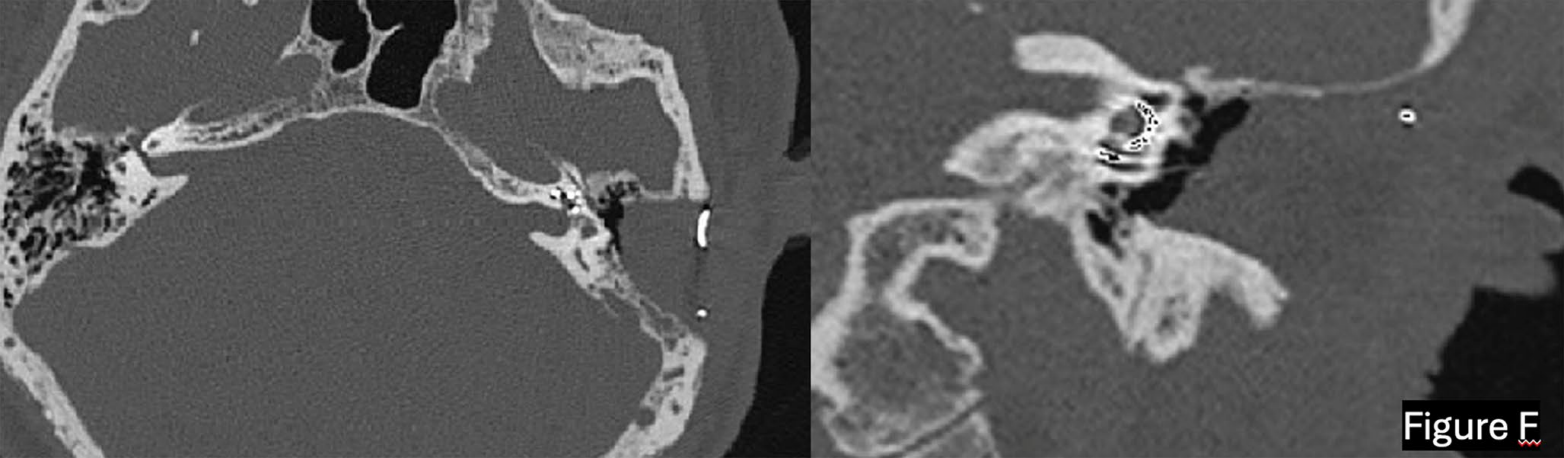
In this post-operative high-resolution CT (HRCT) scan is visible an example of the electrode array placed correctly
